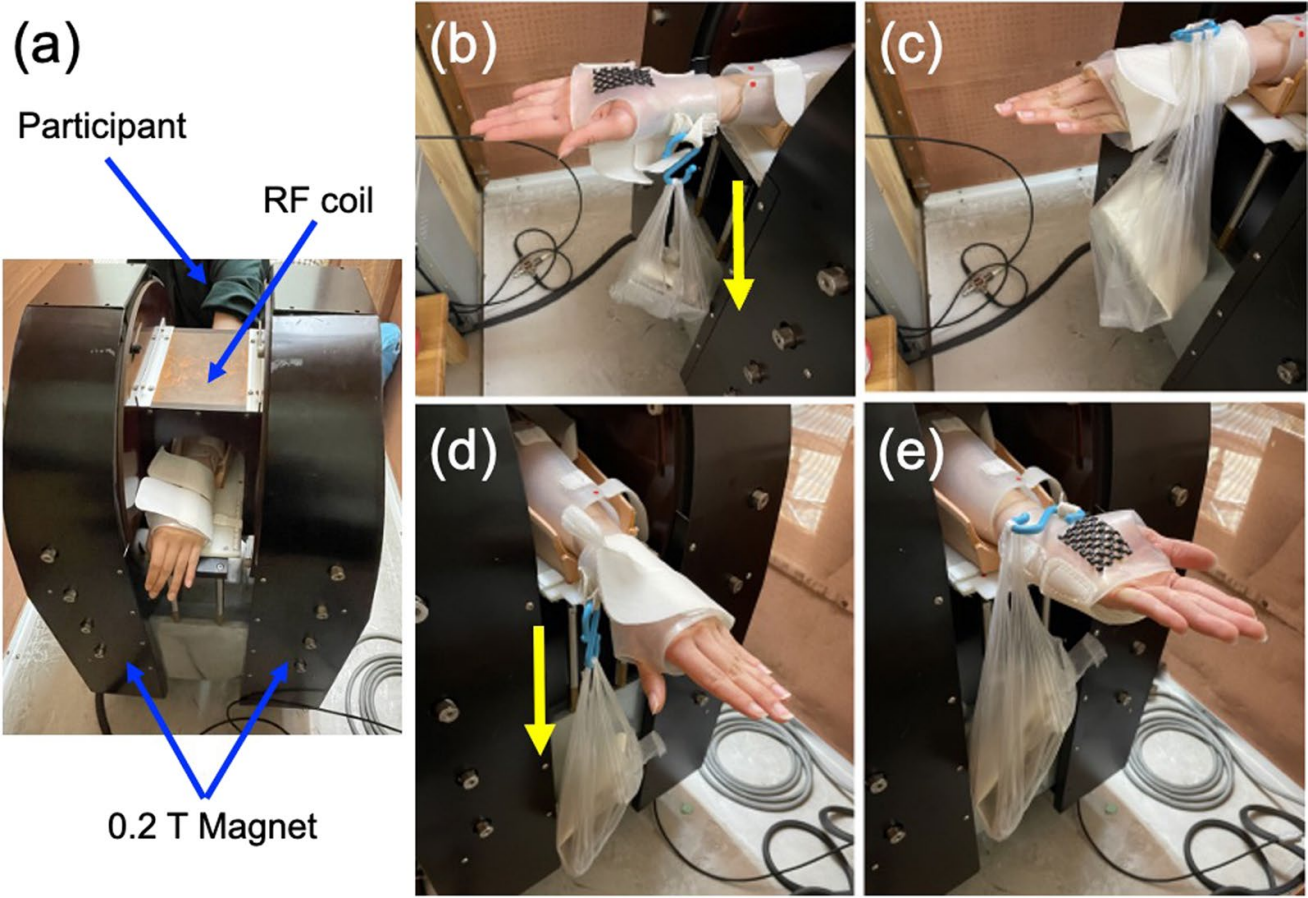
The MRI experimental setup. a Top view of the 0.2 T permanent magnet, a^1^H solenoidal RF coil, and position of participant with his/her forearm during MRI measurement. b, c The cuff setting employed for the supination exercise. The cuff was composed of an opaque, low density polyethylene material, and a weight (5, 15 or 25% of the MVC) was attached on the palmar side of the cuff. The initial position of the hand was set in the fully pronated position (b). The forearm supinated against the weight, and the participants kept the fully supinated position for 1 s (c), then, returned passively to the fully pronated position. This exercise was repeated at 2 s intervals until the participant was unable to continue the forearm rotation. d, e The cuff setting employed for the pronation exercise. A weight was attached on the opisthenar side of the cuff. The initial position of the hand was set in the fully supinated position (d). The forearm pronated against the weight, and the participants kept the fully pronated position for 1 s (e), then, returned passively to the fully supinated position. The yellow arrows show the gravity direction
